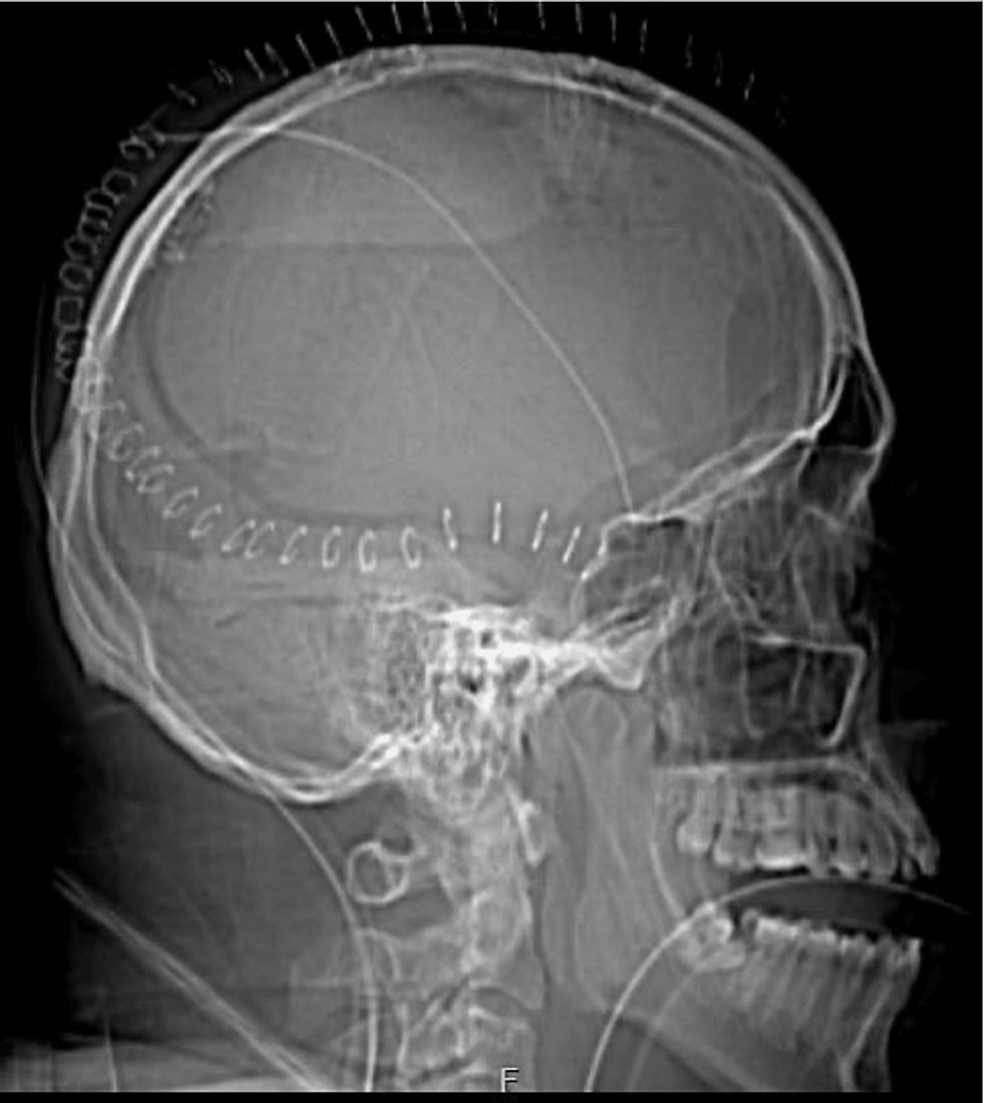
Lateral CT scout image showing large craniotomy bone flap with cranial fixation plates along the medial aspect to allow for the hinge. Image courtesy Dr. Salazar Jones.
